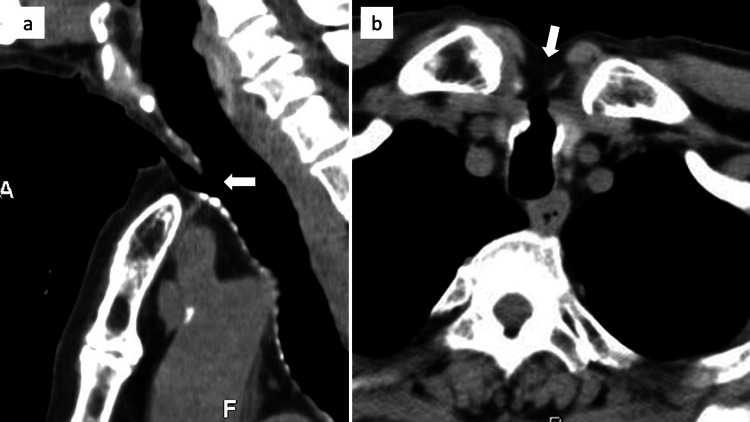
Preoperative computed tomography (CT) findings: (a) sagittal view; (b) axial view. A tracheal defect with a size of approximately 10 mm was observed caudal to the sternal notch, as indicated by the arrow.
